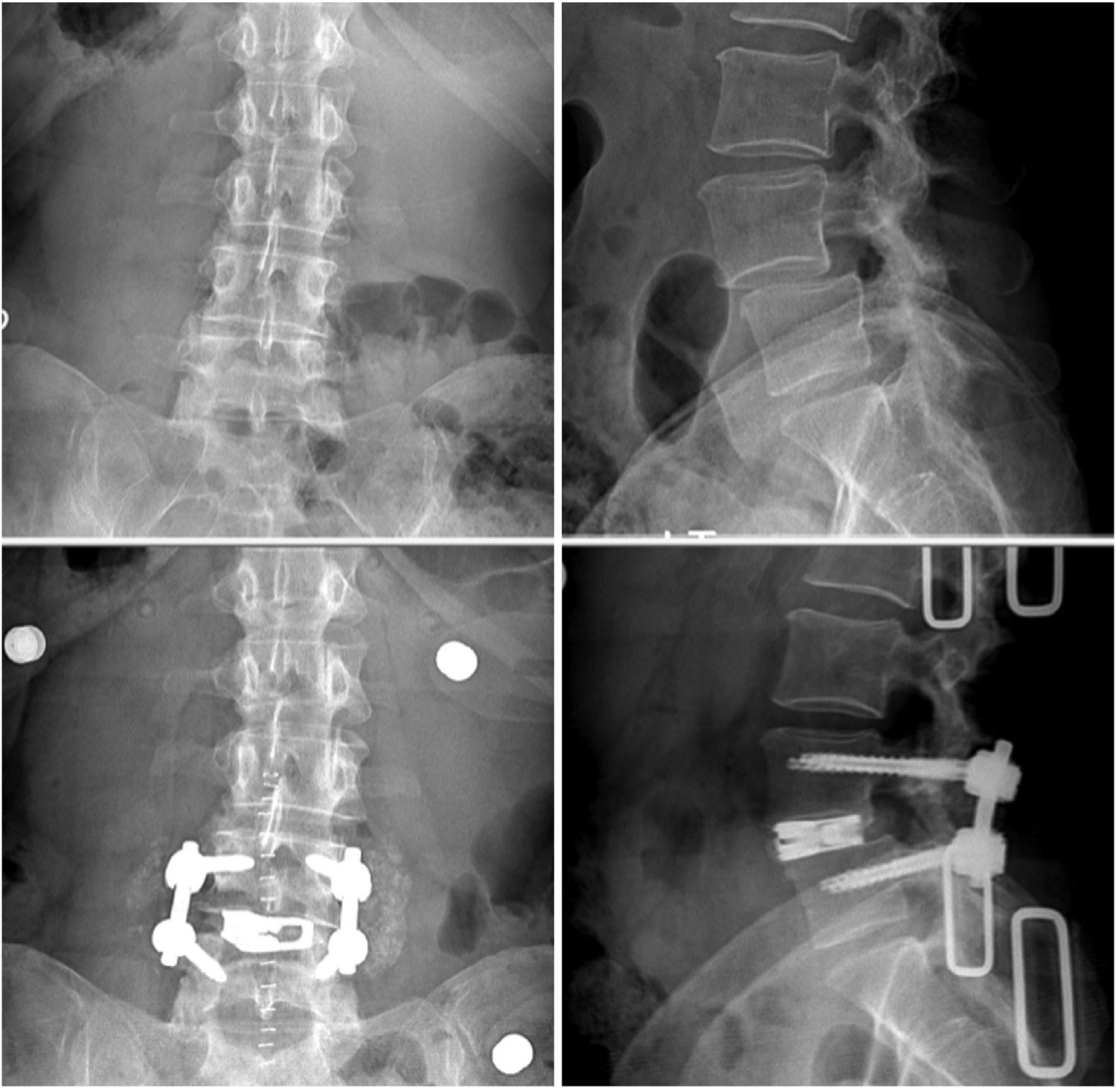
Pre- and postoperative pairs of radiographs showing the device placed at the L4/5 level.
